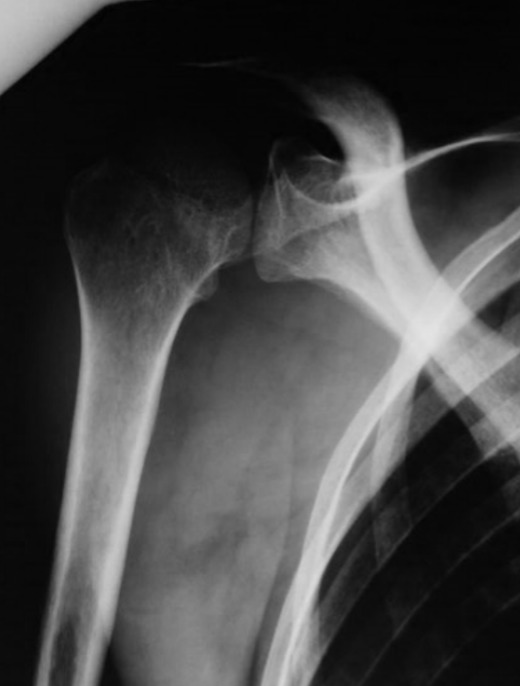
Radiographie standard de l’épaule (incidence de face), une déminéralisation osseuse péri-articulaire
